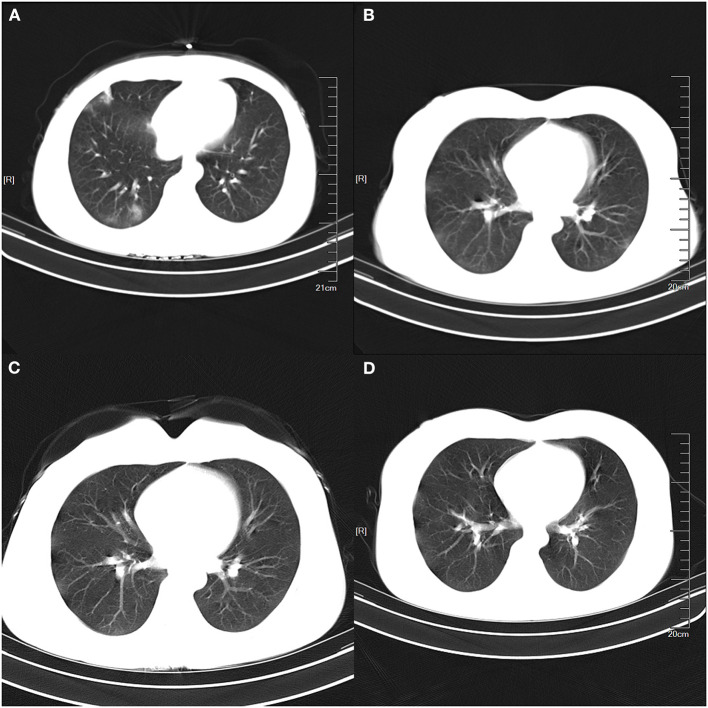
The specific Chest CT scans of the re-positive patient at different periods. (A) was the multiple patchy high-density shadow on bilateral lungs at admission; (B) was the patchy low-density shadows at first discharge, which was significantly better than that of admission; (C) showed patchy low-density shadows in the right lower lobe at the first follow-up visit; and (D) showed no abnormal high-density shadows at second discharge.
